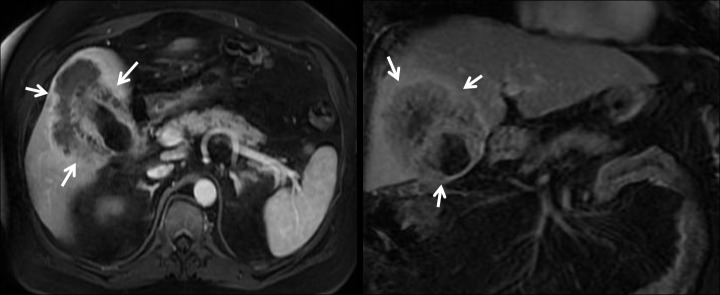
Magnetic resonance imaging (MRI) of the liver showing mass in relation (arrows) to portal vessels.
